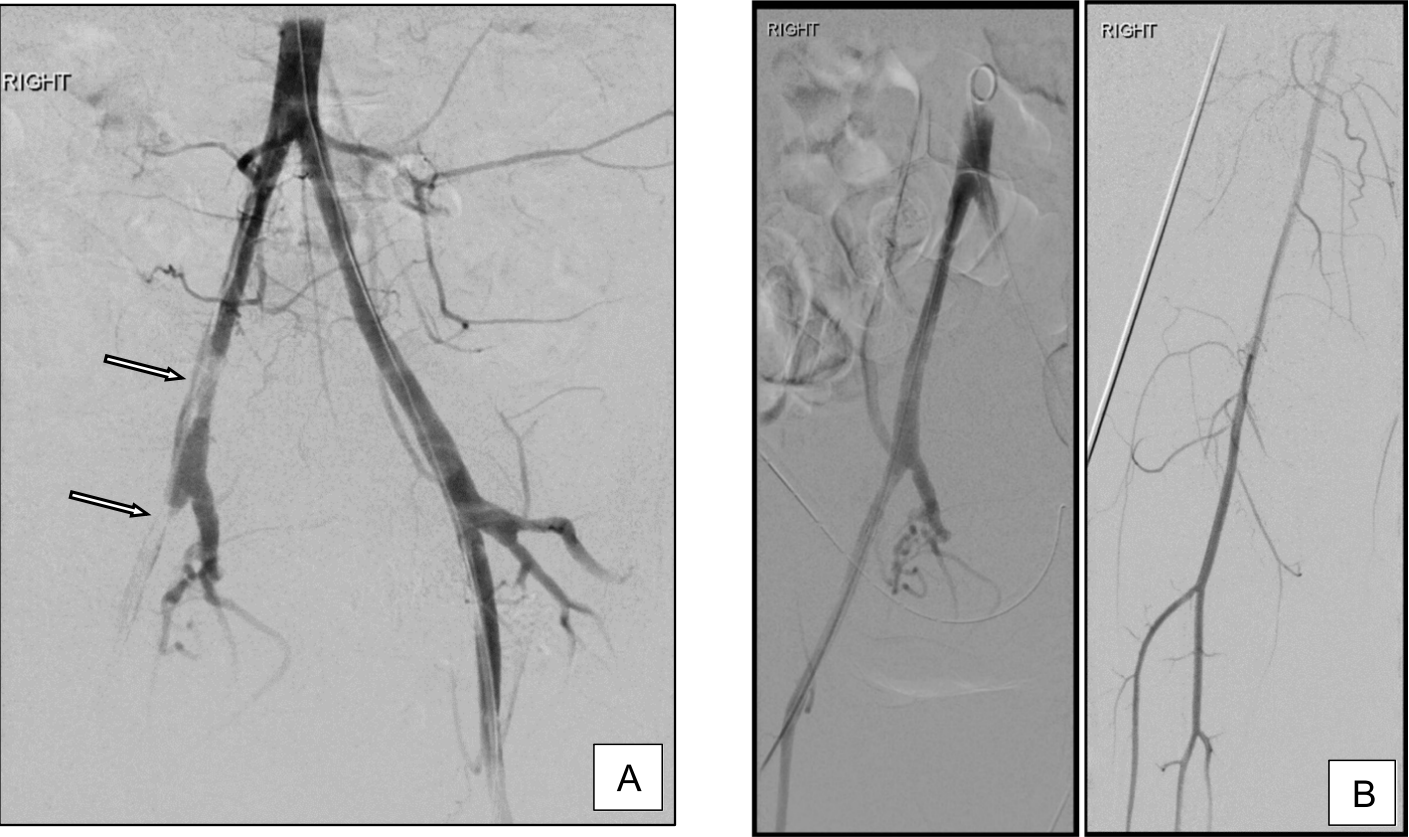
A Angiography prior to sheath removal demonstrates thrombus within right common iliac and external iliac arteries (arrows). Patient remained asymptomatic with warm, well-perfused leg throughout. B Patent arteries following aspiration thrombectomy. Run off vessels all intact. Leg monitored continuously and duplex ultrasound subsequently confirmed resolution of thrombus. No adverse sequelae
